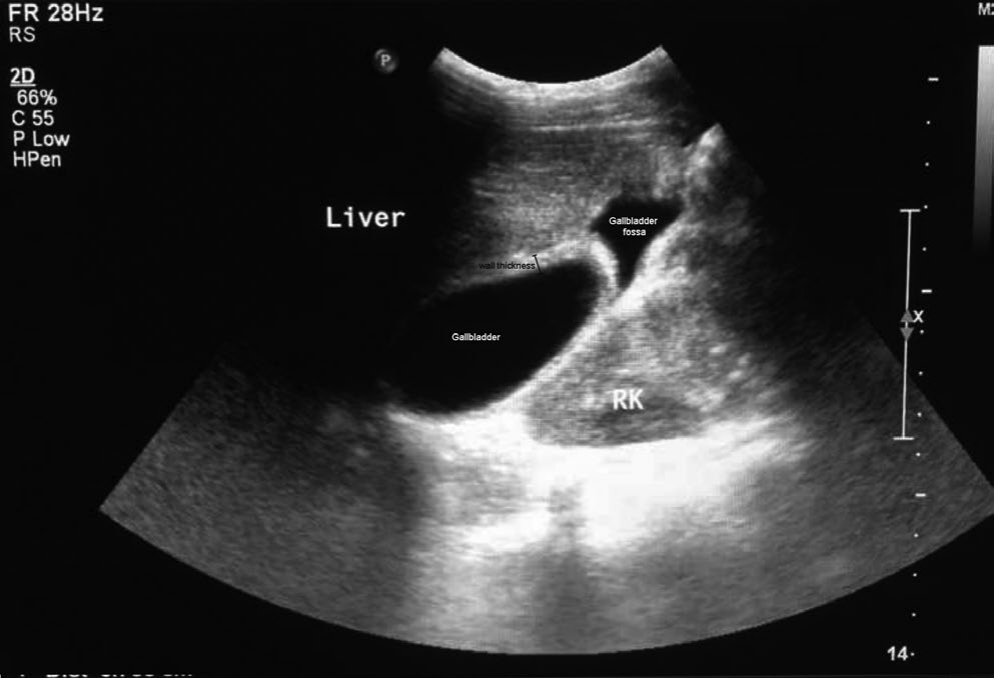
Ultrasound appearance of gallbladder volvulus: gallbladder fossa marked with an arrow (↑), note that gallbladder is floating out of fossa/anterior
